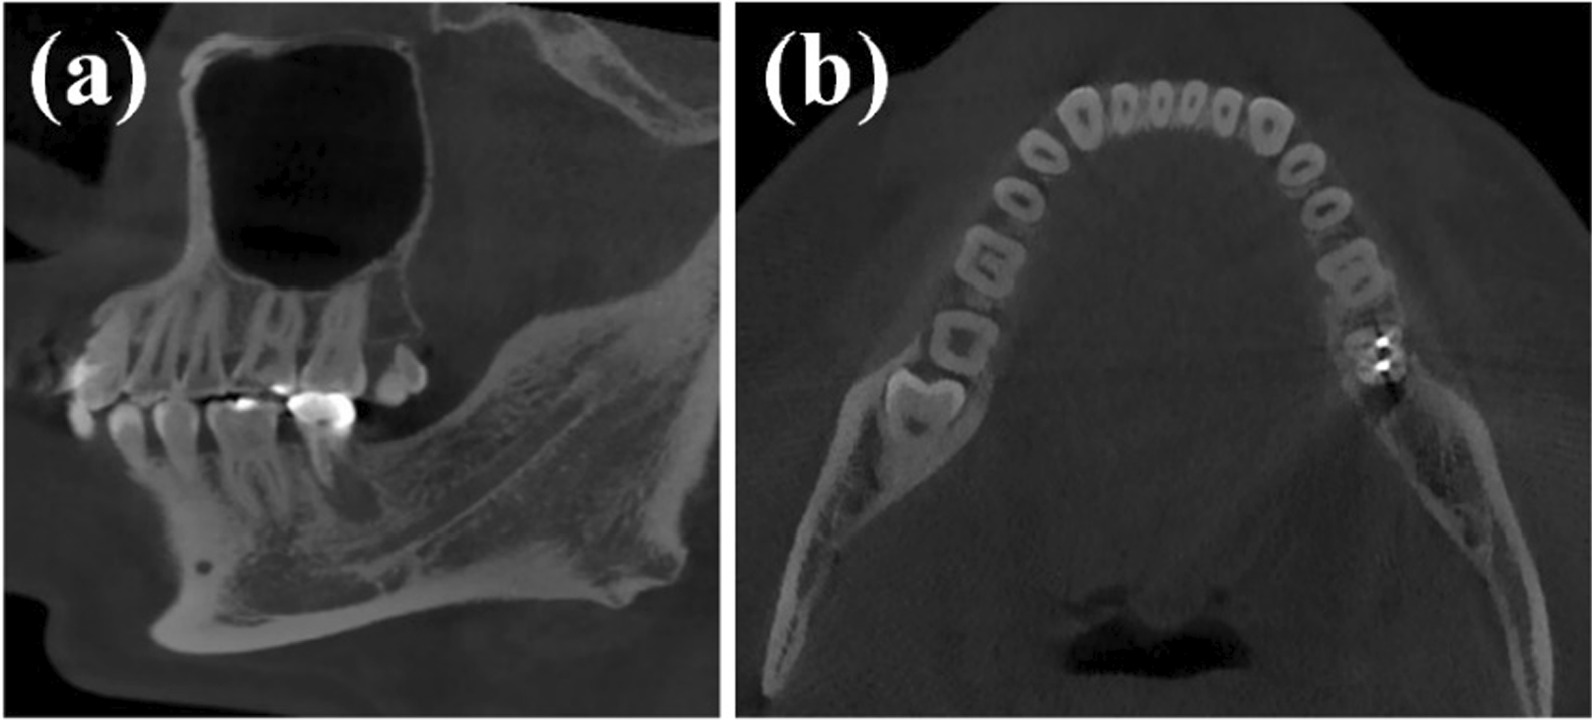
a Periapical inflammation in CAD/CAM zirconia post in mandibular molar, b root fracture in CAD/CAM zirconia post in mandibular molar
